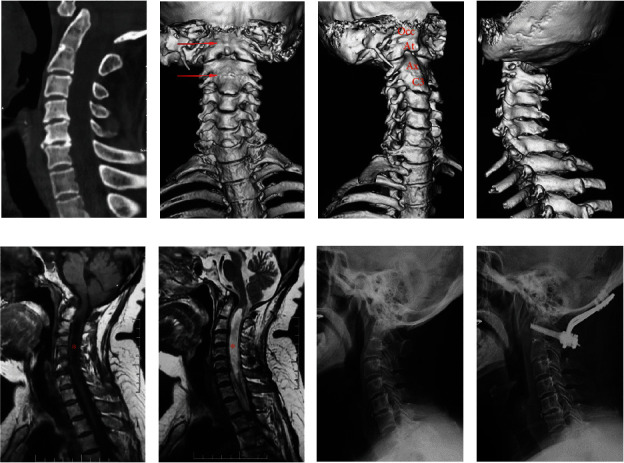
One 62-year-old woman (patient ID 22KAH0010) with quadriplegia carried multiple nonsynonymous mutations in *MEOX1* and *KMT5A*. (a–d) the radiological imaging showed sandwich fusion that is occiput-atlas fusion combined with axis-C3 vertebra fusion. The areas pointed out by the red arrows in b are the fusion sites. (e, f) MRI showed AAD compressing the spinal cord as well as a huge syringomyelia where the red pentastars indicate. (g) Preoperative lateral X-ray films showed sandwich fusion, AAD, and swan neck deformity. (h) Postoperative lateral X-ray films showed atlantoaxial joint was in anatomical reduction.
